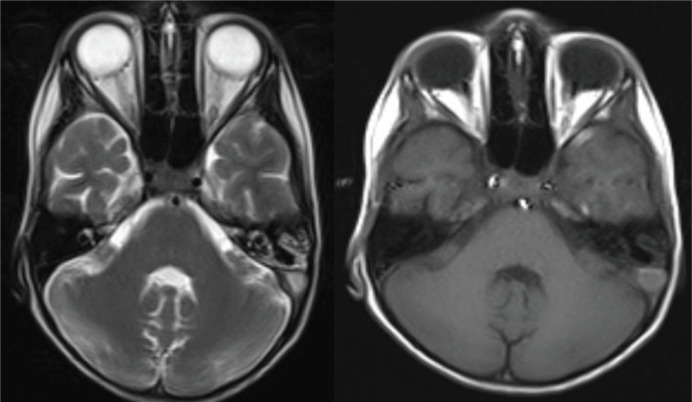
Brain MRI: Abnormal hyperintense signal in left lateral sinus in T1WI & T2WI
